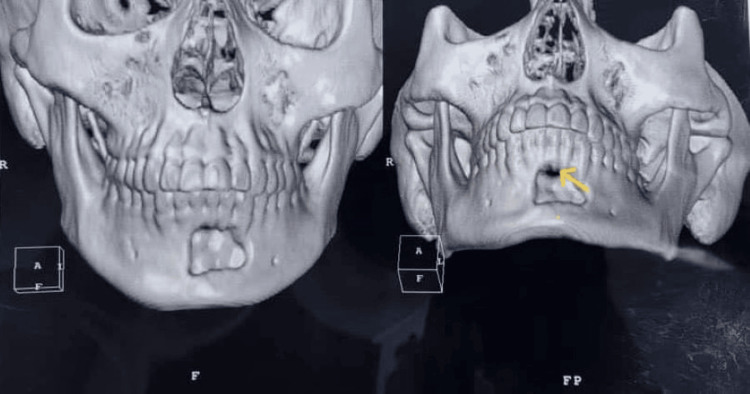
Postoperative CT scan. The scan showed a decrease in the lesion size and new bone deposition (the yellow arrow).
